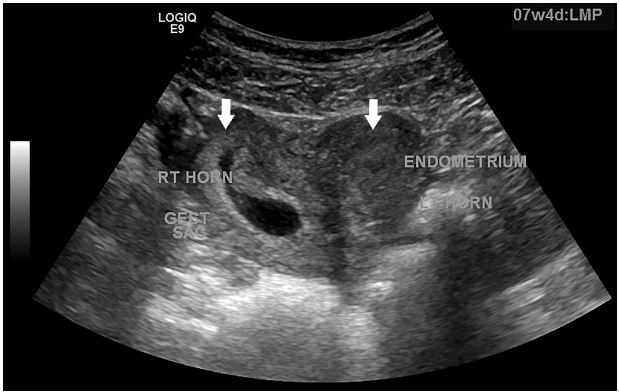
Transvaginal, coronal sonogram showing the classic heart-shape with an intrauterine pregnancy with gestational sac in the right horn (see arrow).
